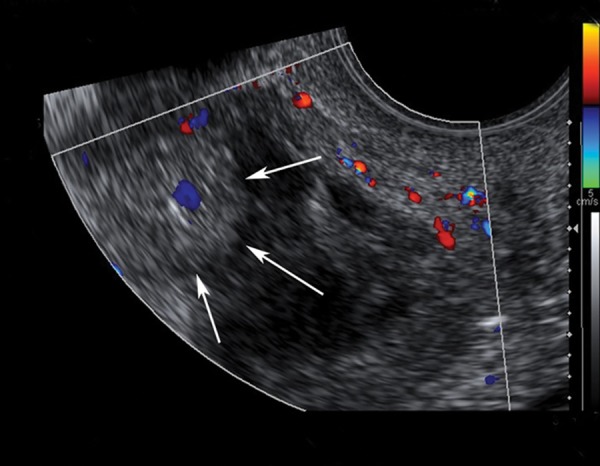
Transvaginal color Doppler interrogation demonstrates a solid mass lesion with smooth contour and a central vascular flow at the anterior wall of the uterine isthmus.
